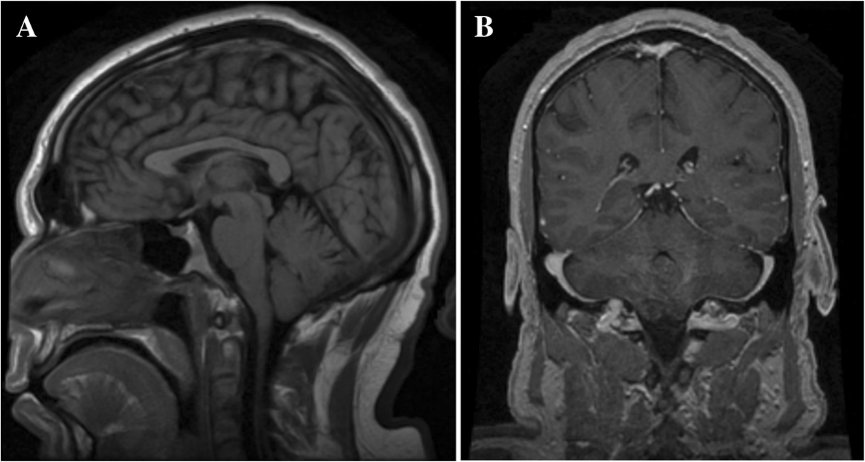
Magnetic resonance imaging of the brain. Chiari malformation type I. (A) Sagittal T2 fluid-attenuated inversion recovery sequence. (B) Coronal spoiled gradient recalled echo (SPGR) post-contrast sequence.
